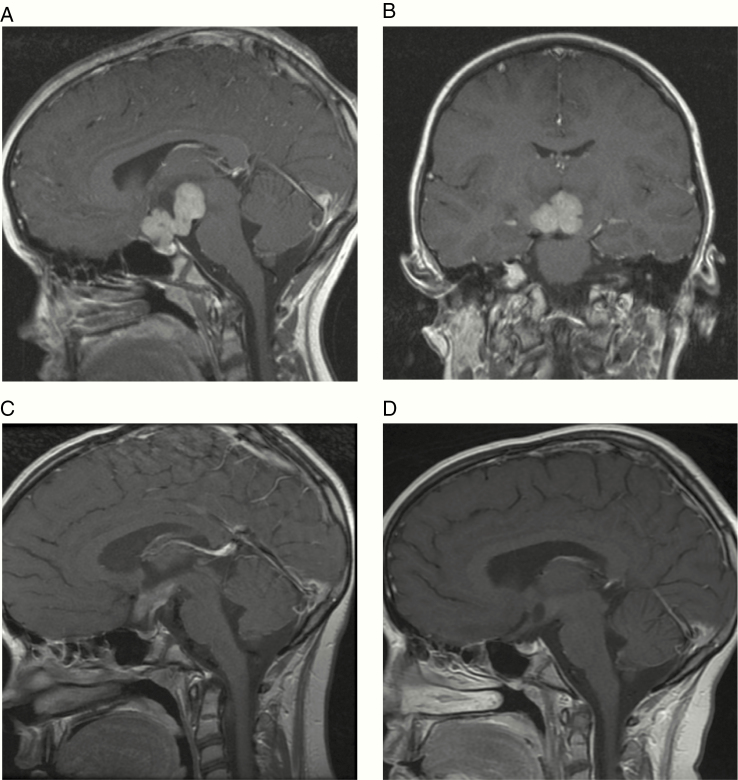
MRI of Case 1 (suprasellar germinoma). (A and B) representative sagittal and coronal views, respectively, at the time of presentation. (C) sagittal view after 2 doses of weekly vinblastine showing dramatic tumor response and (D) sagittal view after completion of vinblastine monotherapy and prior to definitive radiotherapy showing further tumor response.
